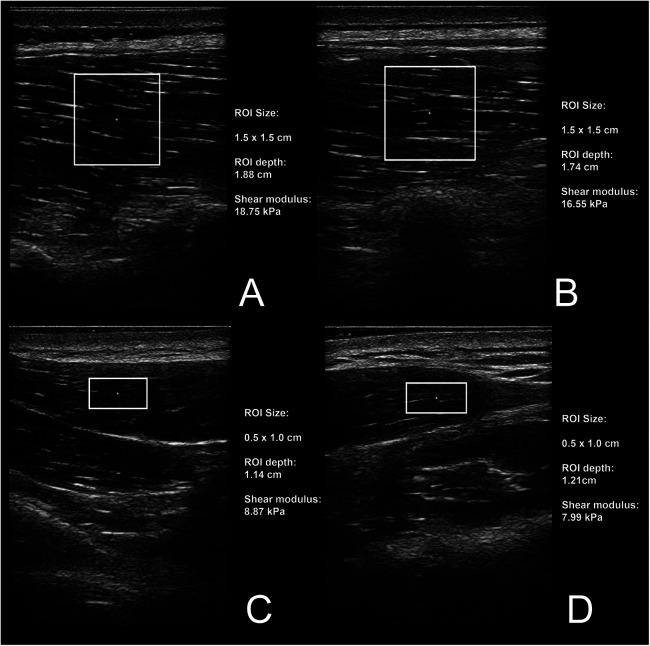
Snapshots of ultrasound measurements for superior (A) and inferior (B) location on erector spinae muscle, and proximal (C) and distal (D) point on upper trapezius muscle.
